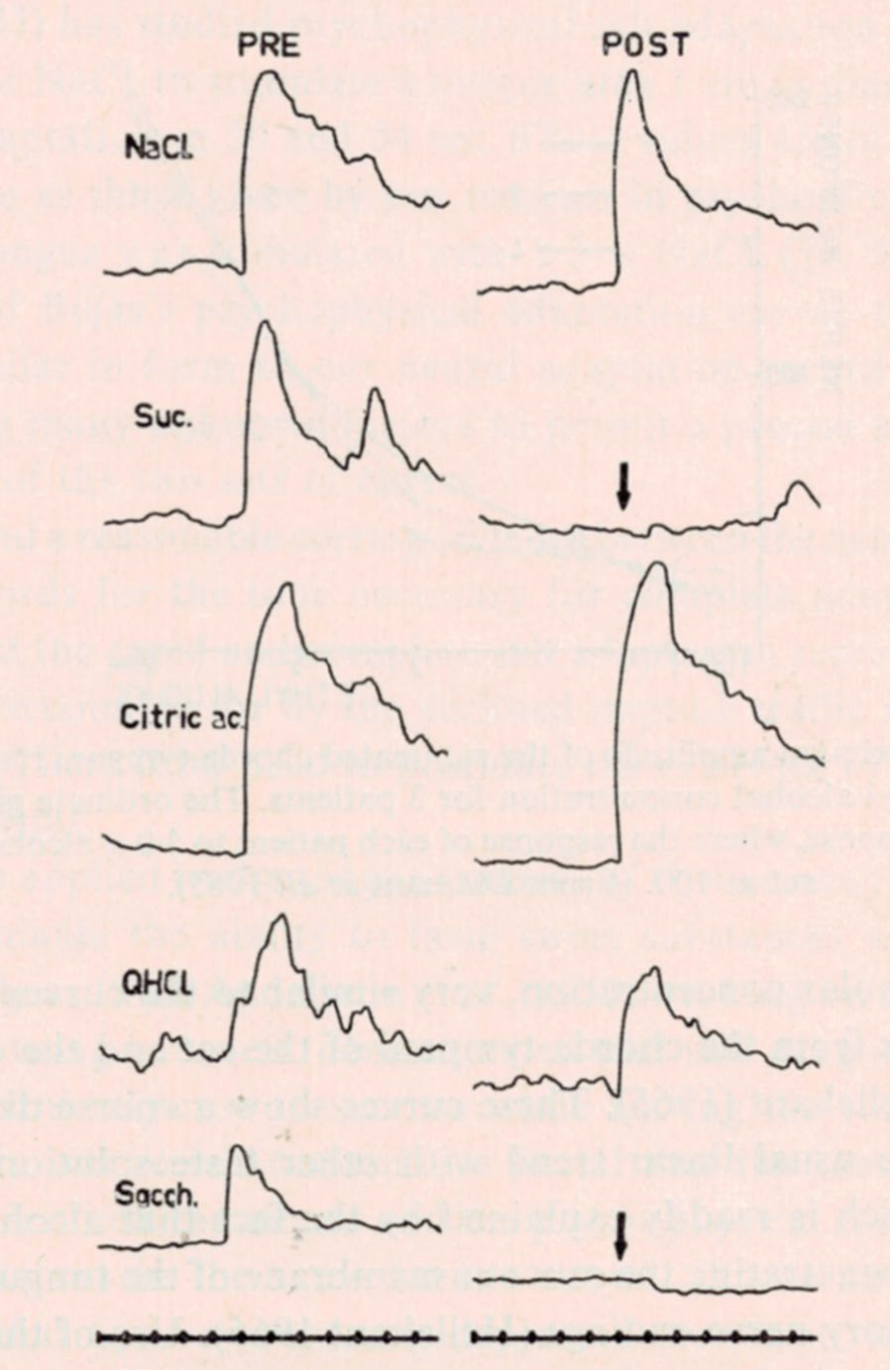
Summated recording from whole CT nerve in the human pre- and post-GA. Time marks in sec. The arrows show the onset of stimulation. [28]
